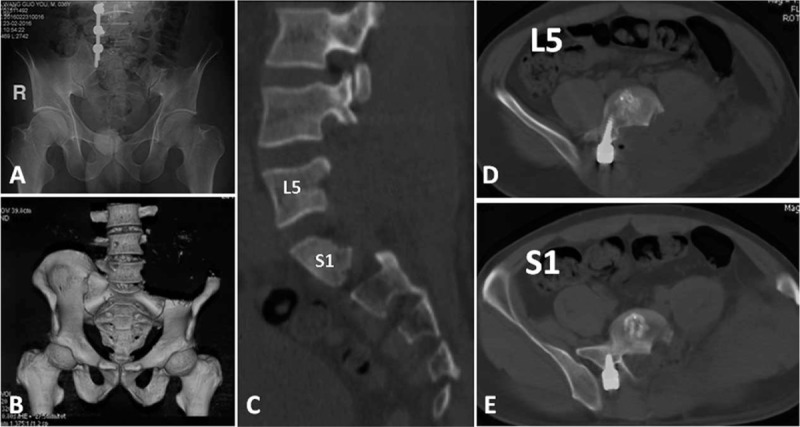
(A) Postoperative anteroposterior radiograph, (B) 3-dimensional computed tomography scan, and (C) sagittal and (D, E) axial images showing the bone resection extent and instrumentation position.
